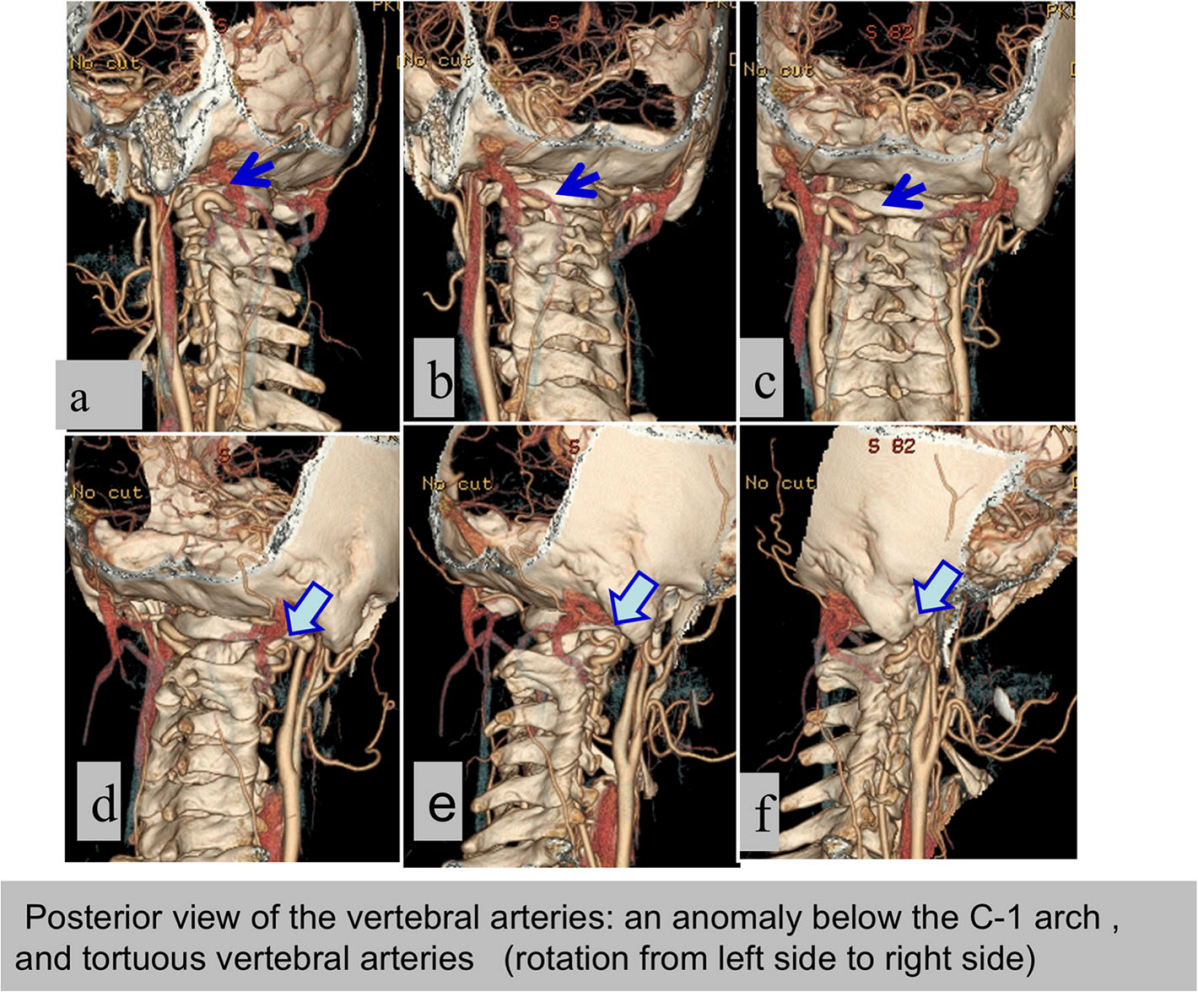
Anomaly of vertebral arteries on CTA. a, b and c: the left lateral, left posterior oblique and posterior view of the VA; the blue arrow showed left VA was tortuous and anomalous riding under the C1 arch. d,e&f: the right lateral view of the VA; the vacant arrow showed right VA was also tortuous under the C1 arch
